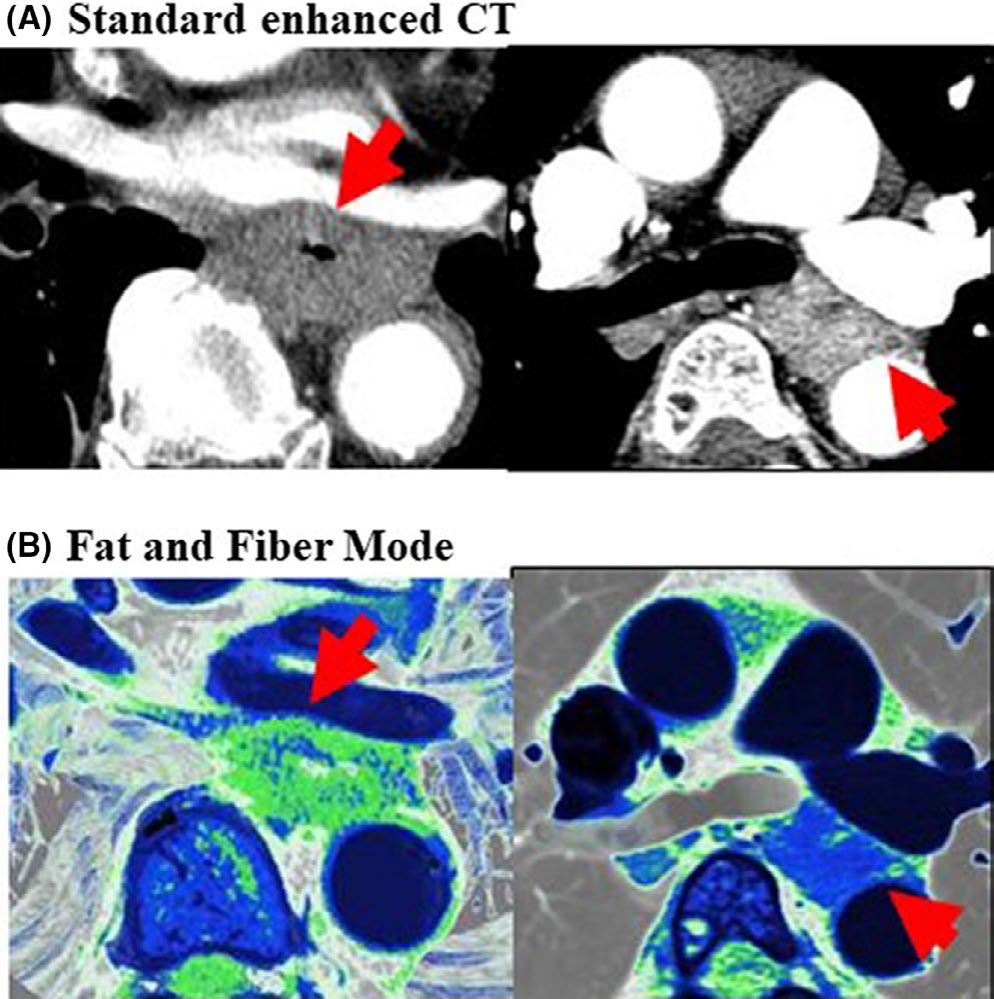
Representative CT images for clinical staging in esophageal cancer. The differential diagnosis between T4 and T3 tumors in esophageal cancer by fat and fiber mode. (A) Standard enhanced CT, (B) Fat and fiber mode
